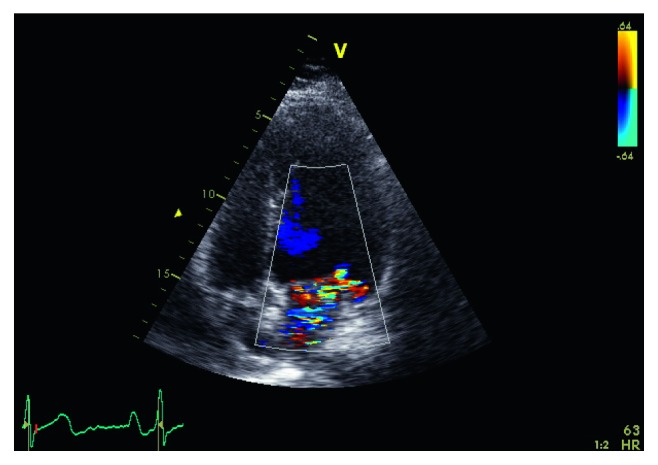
Echocardiogram showing severe mitral regurgitation.
